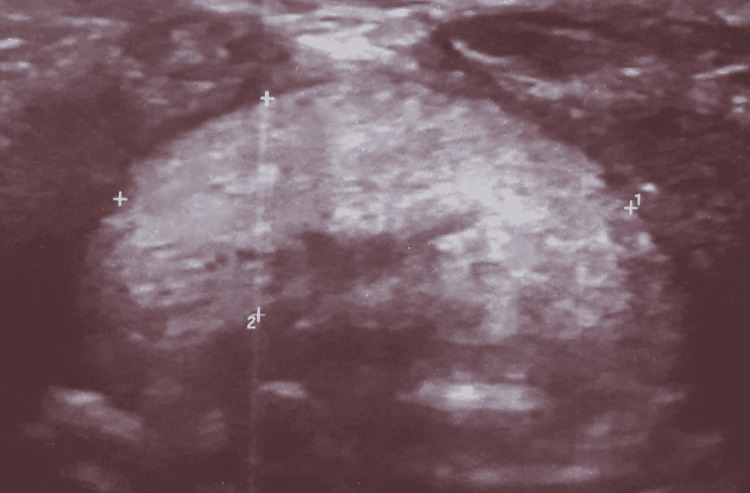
Ultrasound image showing the ectopic thyroid Ultrasound image in a transect through the upper limit of the hyoid bone shows a well-limited tissue structure, with a coarse oval shape, hyperechoic in relation to the homogeneous muscles, reminiscent of the thyroid parenchyma. It should be noted that the thyroid cavity was empty on ultrasound examination. Arrows 1 and 2 allow the estimation of the volume of the ectopic thyroid. It measures 21 × 9 mm.
